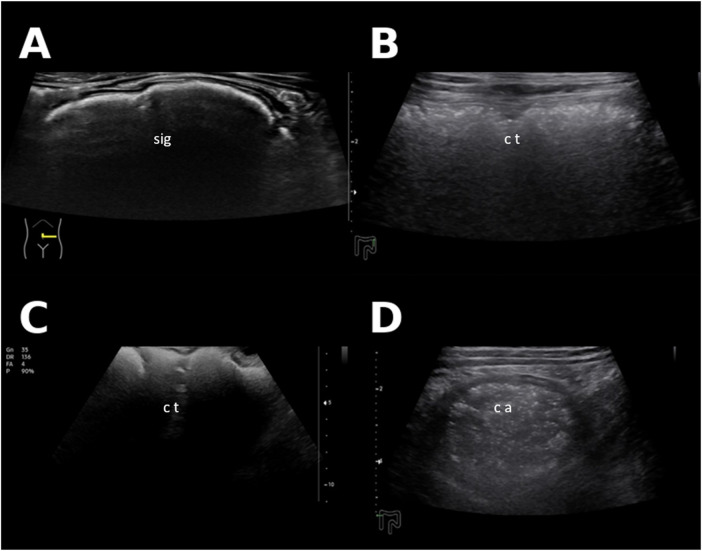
Representative transabdominal ultrasound appearances of colonic contents in children: (A) hard stool with strong anterior echoes and posterior shadowing; (B) soft stool with weak echoes and reduced shadowing; (C) intraluminal gas with reverberation artifacts; (D) liquid stool with a mixed “mousse-like” pattern (sig: sigmoid colon; ct: transverse colon; ca: ascending colon).
